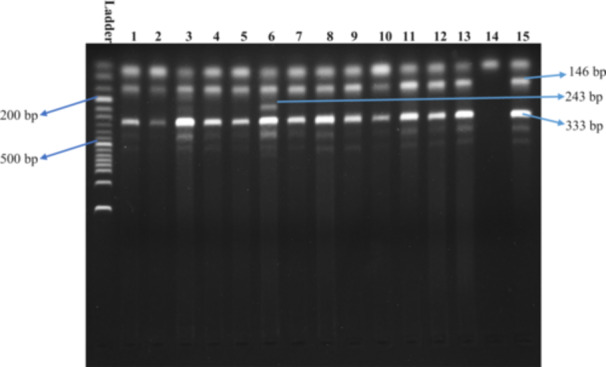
PCR amplification bands of CC, CT, and TT genotypes for SNP rs2285053 and product sizes were 146 bp for C allele, 243 bp for T allele, and 333 bp for the control band. The first lane represents a 50 bp DNA ladder; lanes 1–5, 7–13, and 15 indicate CC genotype; lane 6 indicates CT genotype; and lane 14 is blank.
